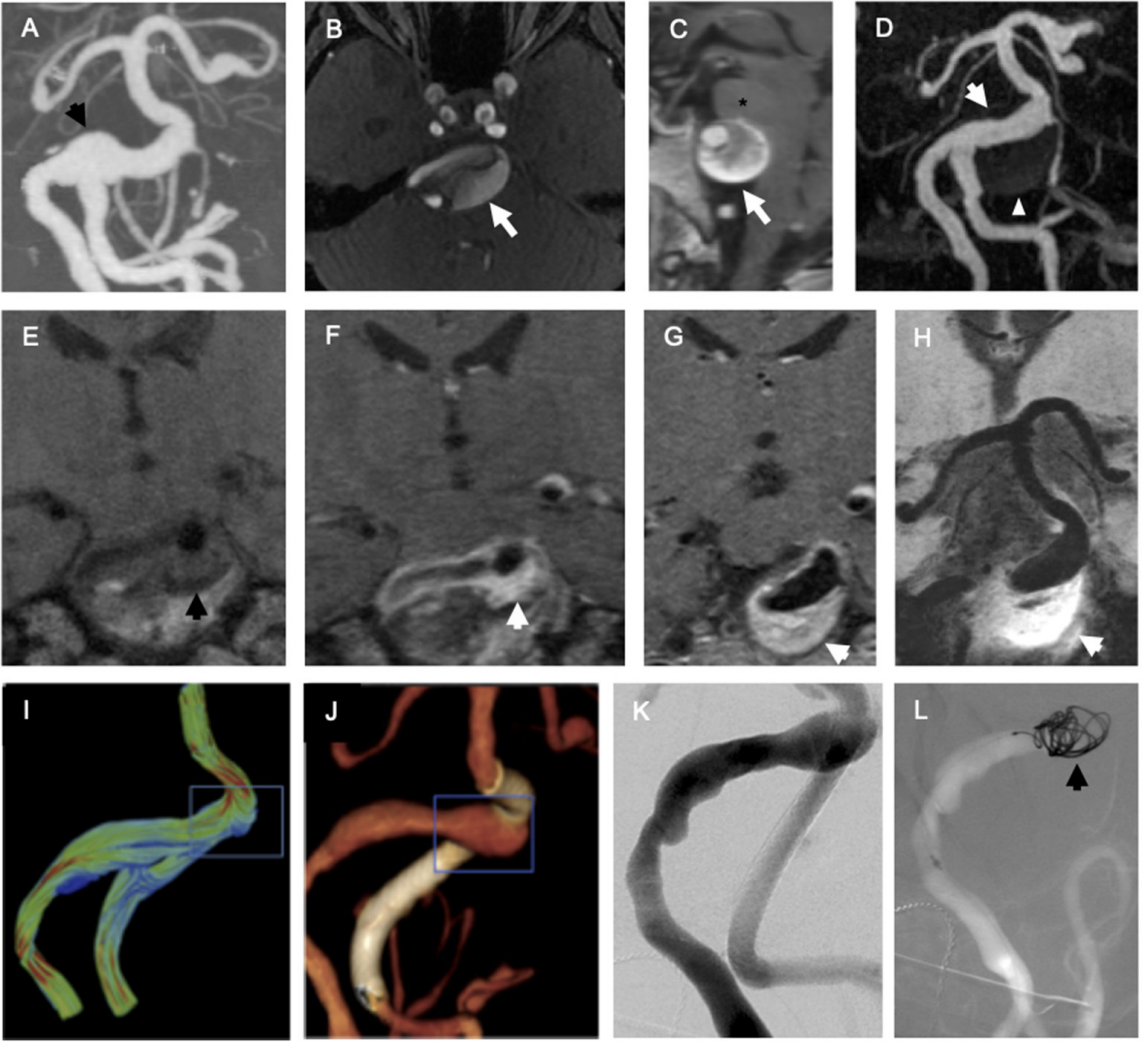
Endovascular biopsy of endothelial cells (ECs) from fusiform vertebrobasilar aneurysm. (A) Coronal maximum intensity projection (MIP) reformat of CT angiogram of the head focusing on the posterior intracranial circulation demonstrates fusiform vertebrobasilar aneurysm with focal dilation of the right vertebrobasilar junction (black arrow). (B) Axial time-of-flight magnetic resonance (MR) angiogram of the head demonstrates fusiform aneurysm involves the basilar artery (white arrow). (C) Sagittal T1-weighted MRI of the brain demonstrates fusiform aneurysm involving the basilar artery (white arrow) compresses the pons of the brainstem (asterisk). (D) Coronal MIP reformat of contrast-enhanced magnetic resonance angiography (MRA) demonstrates fusiform aneurysm involves the basilar artery (white arrow) and the partially thrombosed aneurysm wall is much larger than the white lumen (white arrowhead). Coronal T1-weighted MRI of the right vertebrobasilar junction before (E) and after (F) administration of gadolinium chelate contrast demonstrates intense enhancement of the aneurysm wall, suggestive of non-specific inflammation (black arrow = aneurysm wall before contrast and white arrow = aneurysm wall after contrast). (G,H) Hyperenhancement of the aneurysm wall (white arrow) is seen at the right vertebrobasilar junction on 0.6 mm slice thickness (G) and 10 mm minIP reformat (H) images. (I) A computational fluid dynamic model derived from angiographic data demonstrates high velocity vectors at the superior aspect of the vertebrobasilar junction (blue box). (J) Three-dimensional volume-rendered reformat of flat panel CT angiogram demonstrates focal dilation of the right vertebrobasilar junction (blue box) and flow-diverting stent in the left intradural vertebral artery (white). (K) Planar right vertebral arteriogram in left anterior oblique projection. (L) Roadmap right vertebral arteriogram in the same projection shows a platinum coil (black arrow) in contact with the endothelium of the right vertebrobasilar junction during endovascular biopsy.
